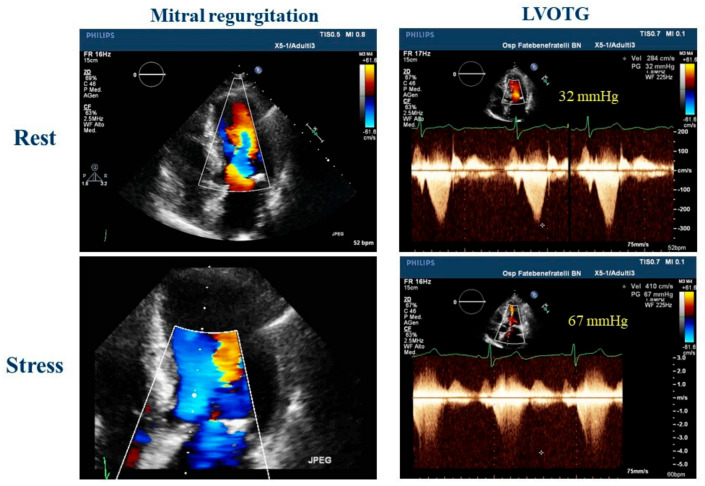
Exercise stress echocardiography in a 51-year-old master athlete, symptomatic for dyspnea during effort and with a family history of hypertrophic cardiomyopathy with a suspicious left ventricular hypertrophy; during effort, is it possible to observe an increase in mitral regurgitation and a significant left ventricle outflow tract obstruction that could explain the dyspnea. LVOTG: left ventricle outflow tract gradient.
